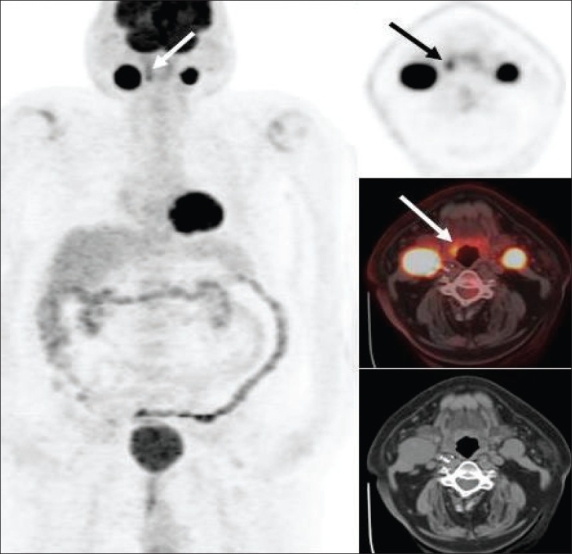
PET (top right), fused PET/CT (middle right) and CT scan (bottom right) images at the level of the tonsils, with an MIP image (on left), from a PET study of a 70-year-old man presenting with a right neck mass. CT scan of the neck revealed bilateral enlarged neck nodes that showed squamous cell carcinoma on biopsy. However, the primary lesion remained unknown after clinical, CT scan and panedoscopic evaluation. The PET scan shows asymmetric tonsillar uptake with slightly more intense uptake in the right tonsil (arrows), as compared to the left. Subsequent biopsy confirmed a grade 2 invasive right tonsillar squamous cell carcinoma
